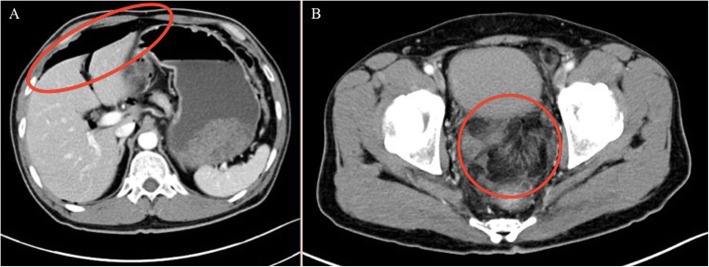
A supine abdominal CT showed diffuse pneumoperitoneum with mainly collected free-air around hepatic flexure (a) and perirectal extraluminal air with fat stranding (b)
